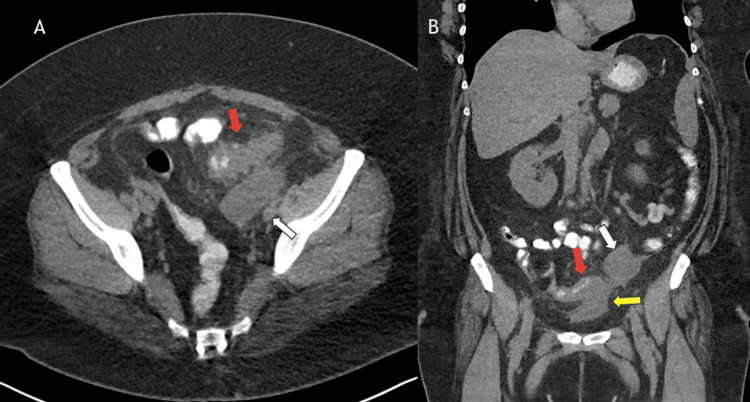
Non-contrast computed tomography (CT) a few slices lower showing all of the findings (2A) Transverse view, (2B) Coronal view White arrow: Round left adnexal lesion with surrounding inflammatory fat stranding. Red arrow: Mural thickening of the sigmoid colon with multiple inflamed diverticula, may be reactive in setting of possible tubo-ovarian abscess or suggestive of diverticulitis. Yellow arrow: Possible inflammatory thickening and continuity between the sigmoid colon and bladder (decompressed with minimal amount of urine) suggestive of colovesicular fistula.
